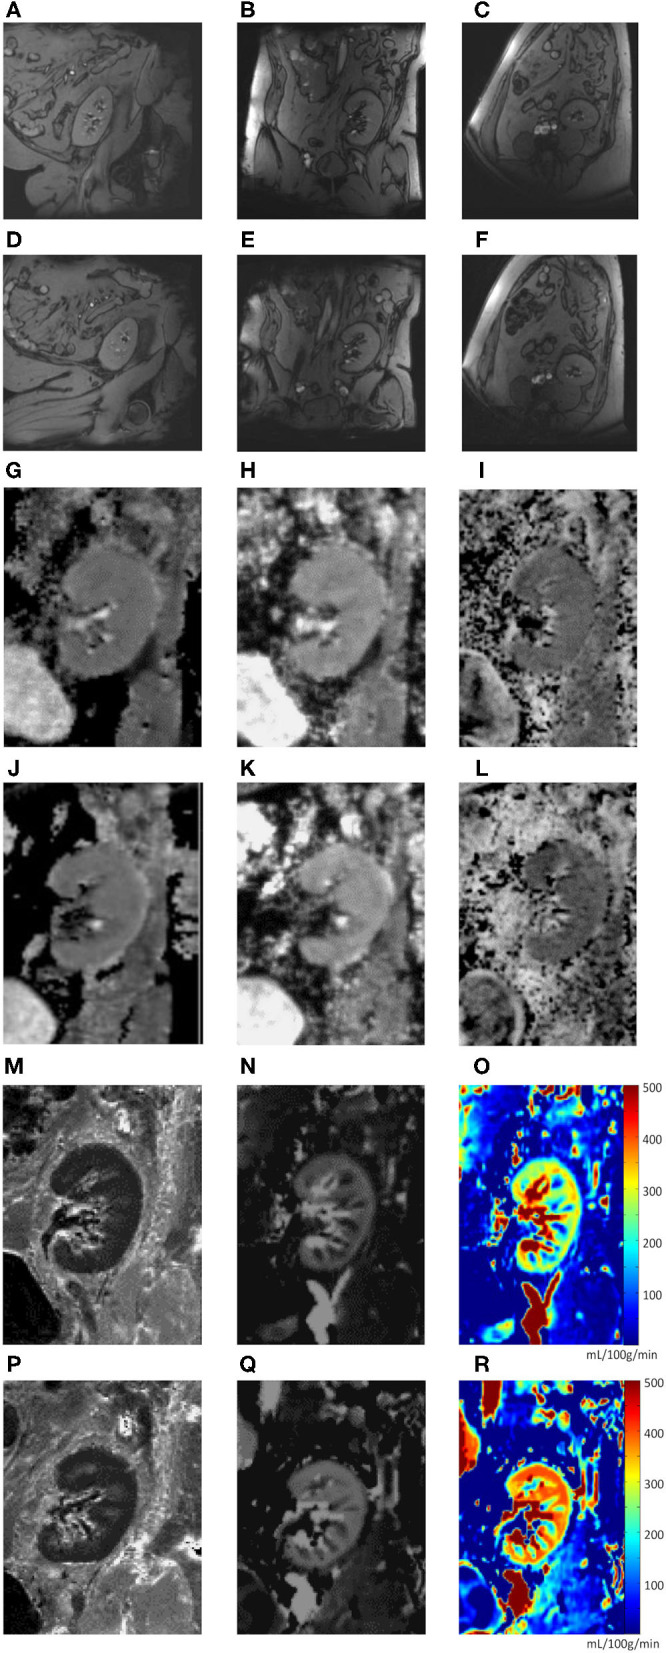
(A–R). Image examples (one subject). Anatomical sequence (T1 map) during neutral hip position (A–C) and hip flexion (D–F) in sagittal (A,D), coronal (B,E), and transverse (C,F) planes. Functional MRI sequences during neutral hip position (G–I, M–O) and hip flexion (J–L, P–R): total apparent diffusion coefficient (ADC_T_) map (G,J); pure diffusion coefficient (ADC_D_) map (H,K); fraction of perfusion (F_P_) map (I,L); resonance transverse relaxation rate (R2*) map (M,P); arterial spin labeling (ASL) map (N,O,Q,R).
